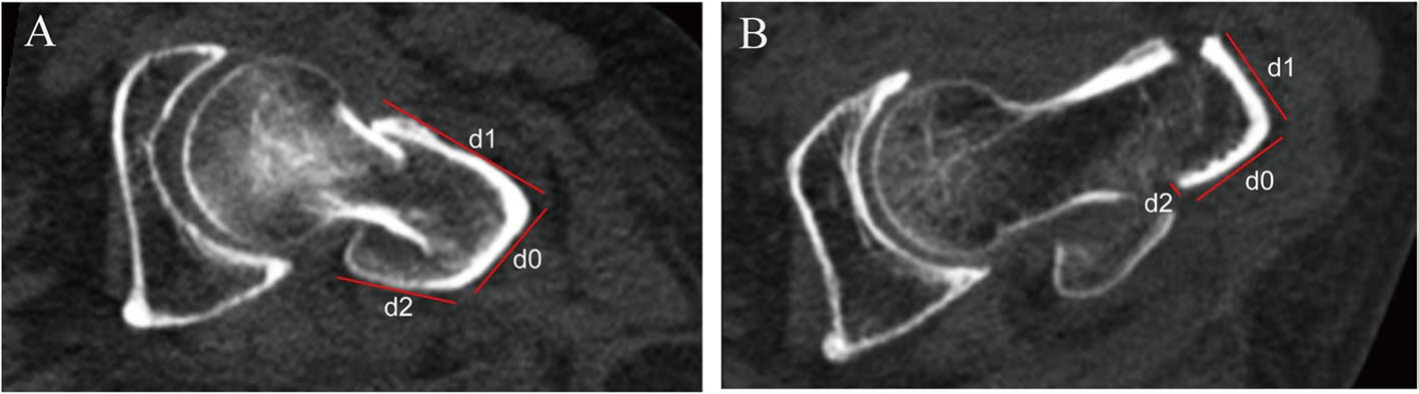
Representative CT images showing the RAC (d1), RLC (d0) and RPC (d2) of the RACL in patients with an A1 fracture (A) and in patients with an A2 fracture (B). The RAC, RPC, and RPC (red line) in A1 fractures were significantly longer than those in A2 fractures. Patients with A2 fractures involved the posterior wall fragment. RAC: remnant anterior cortex, RACL: remnant axial cortical length, RLC: remnant lateral cortex, RPC: remnant posterior cortex
